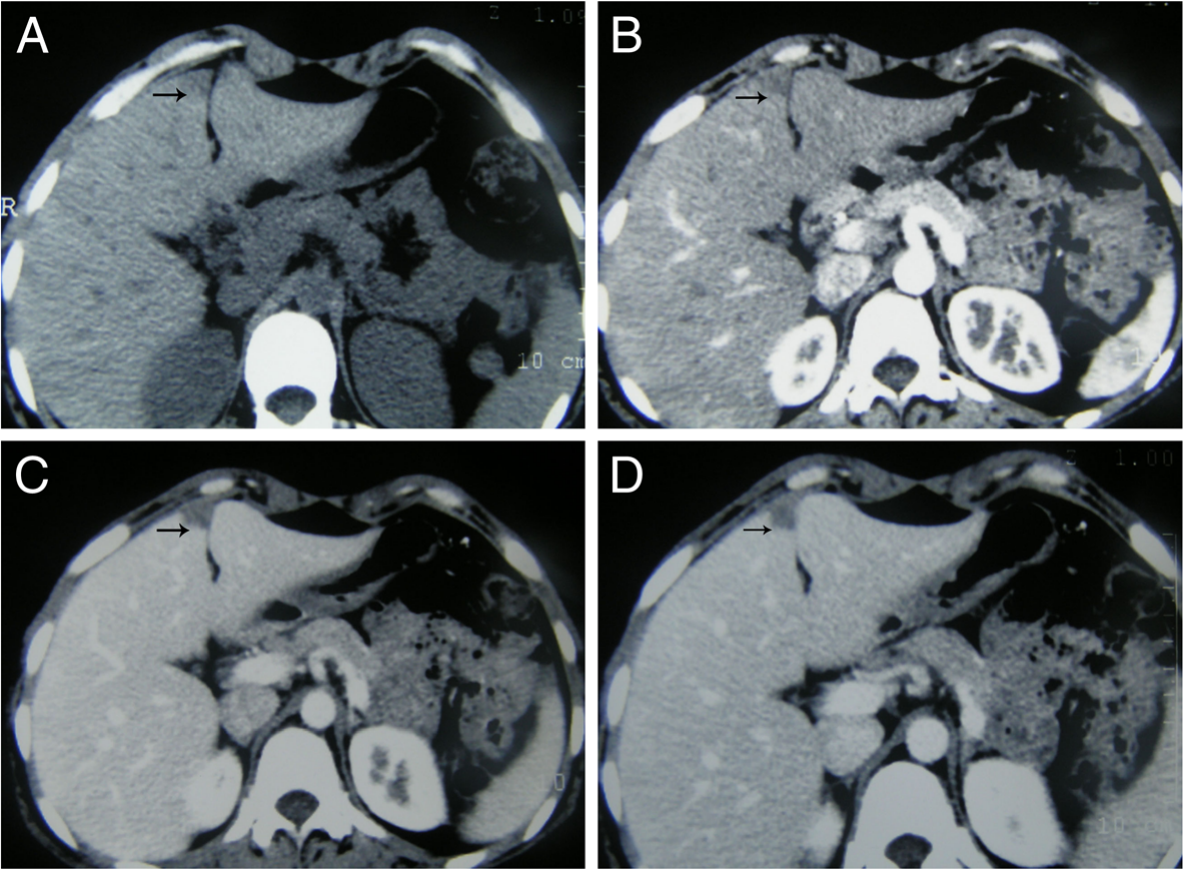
Computed tomography (CT) findings. In each panel, the arrow indicates the pseudolesion. (A) Unenhanced phase CT image. (B) Arterial-dominant phase CT image. (C) Portal-dominant phase CT image. (D) Equilibrium phase CT image.
